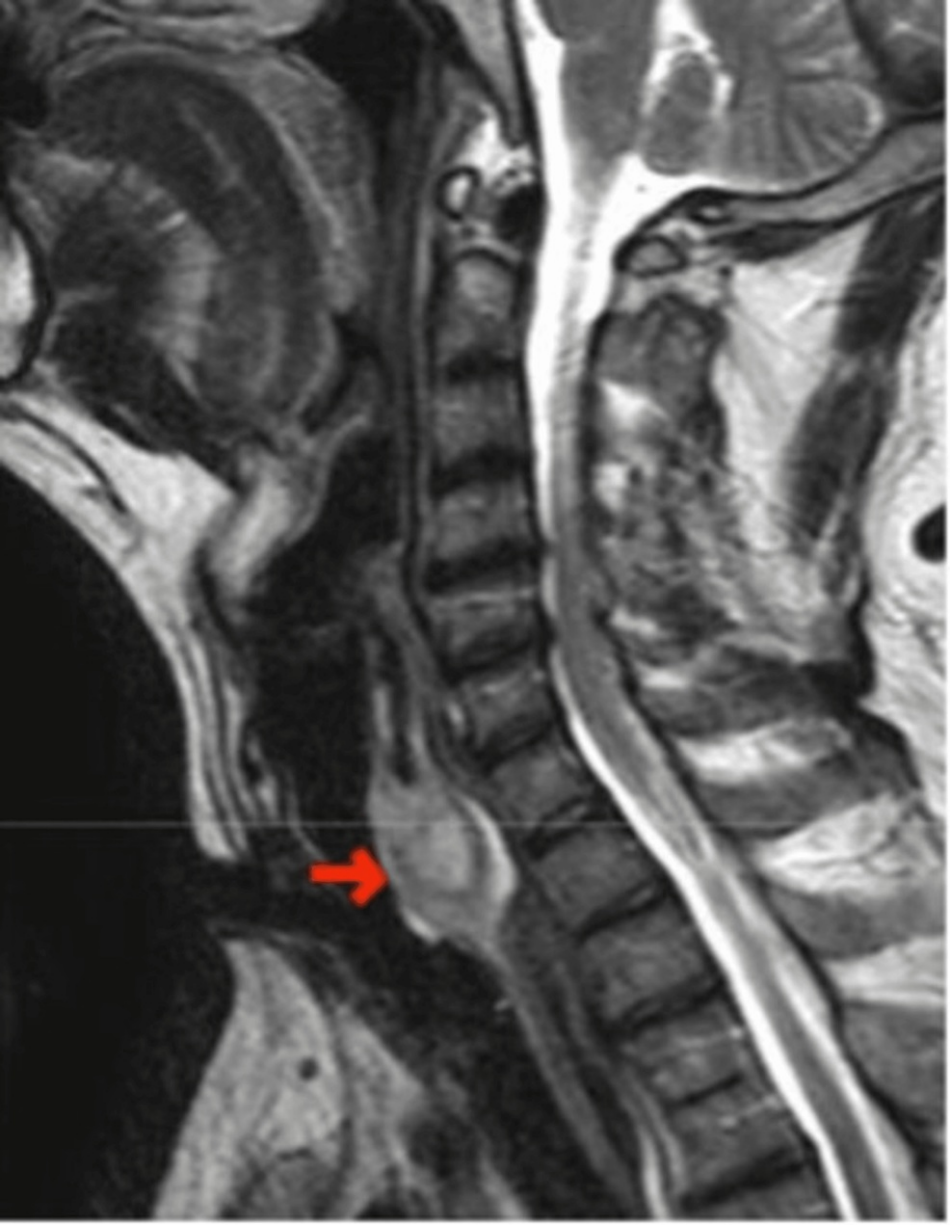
CT sagittal view of the posterior endoluminal tumor located at the upper part of the trachea that invades the posteroinferior perichondrium of the cricoid lamina and displaces the hypopharynx and esophagus
